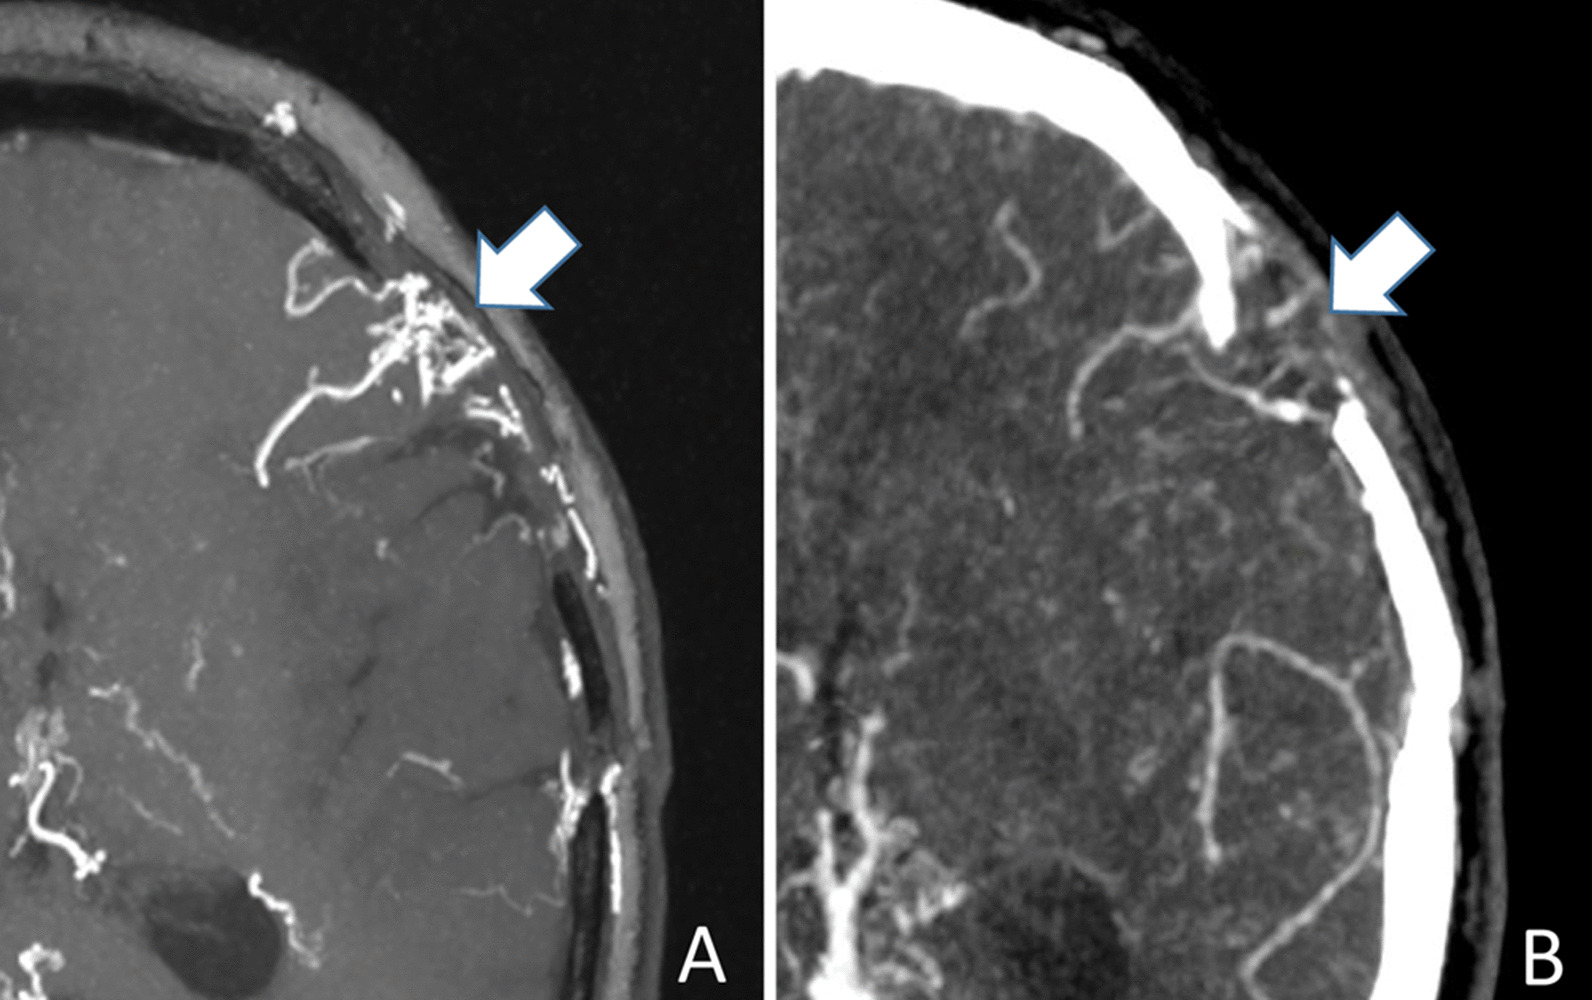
MIP images of a 13-year-old female patient 7 months after surgical revascularization. Collateral formation from neovascularization into the brain pial surface is ranked as Grade-3 on CS TOF-MRA (A), while which is ranked as Grade-2 on CTA (B)
